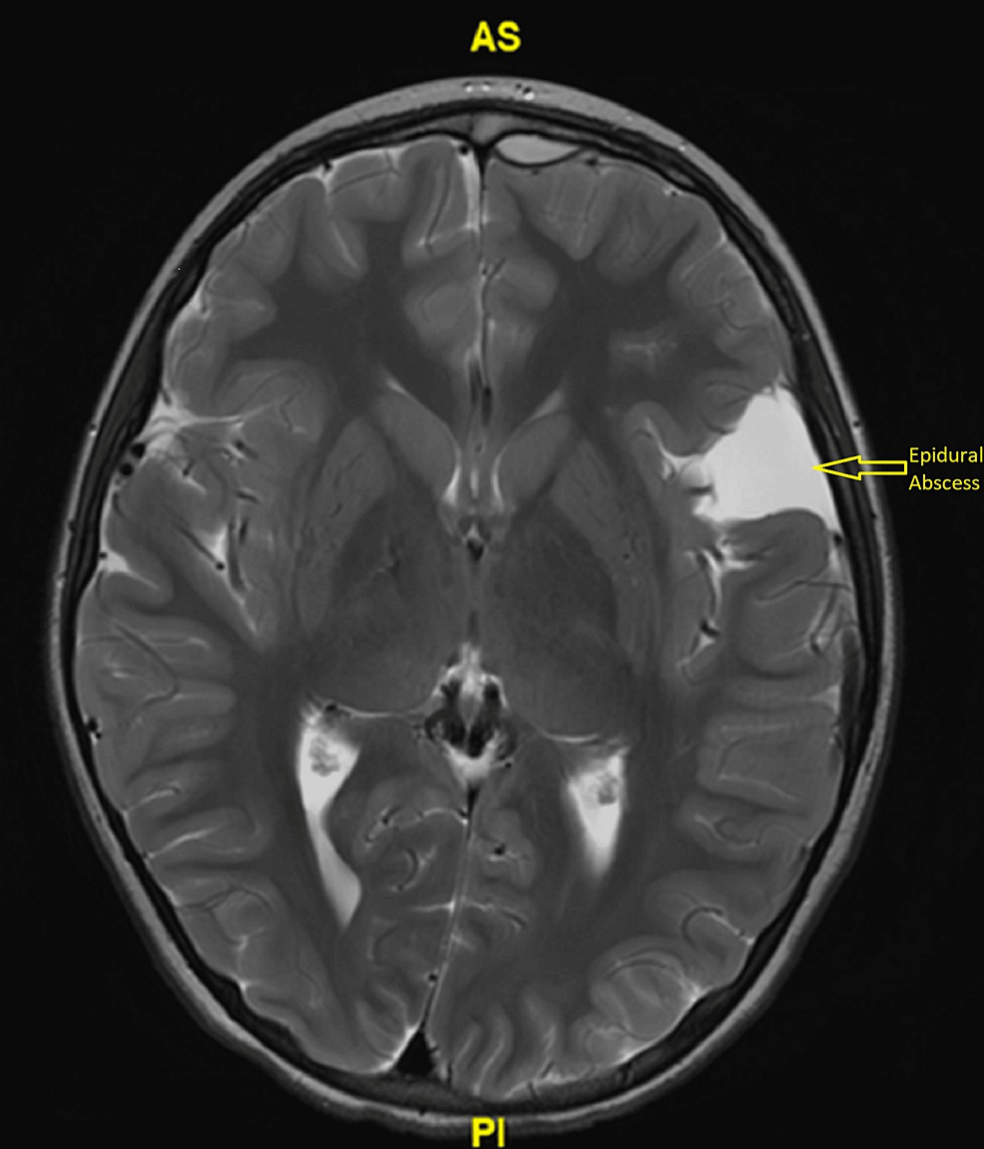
Axial view of brain MRI demonstration small left frontal epidural abscess
